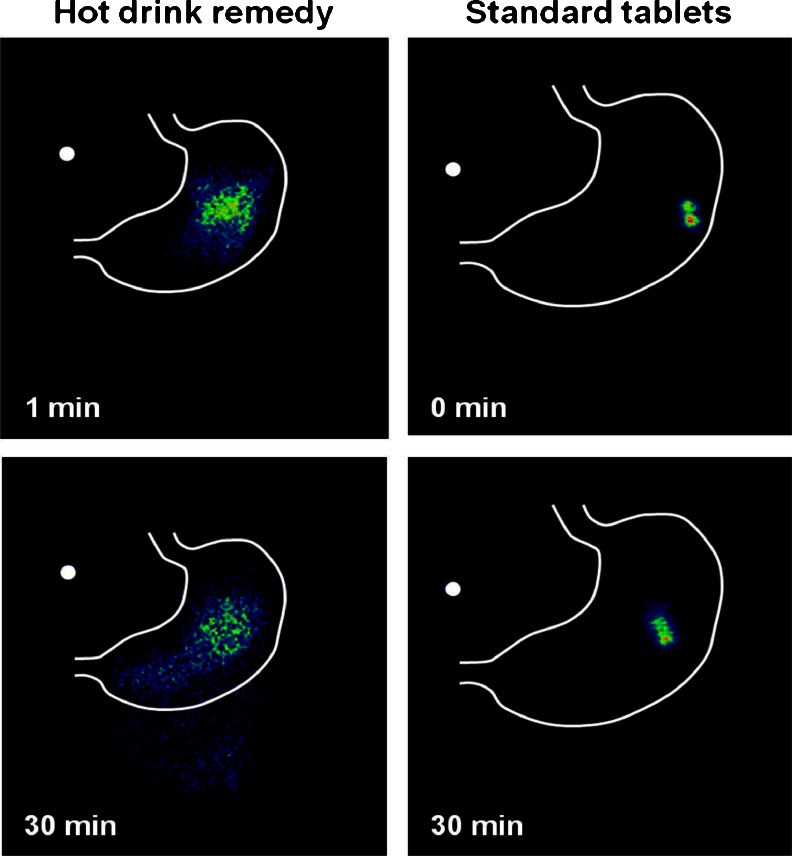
Example scintigraphic images of gastric emptying behaviour of hot drink and standard tablets (exact times recorded for each image taken).
